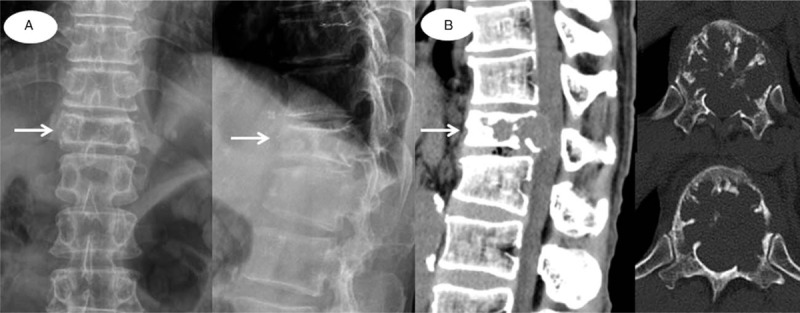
(A) Simple radiographs (anteroposterior and lateral) showed the decreased height of the T12 vertebral body. (B) CT images showed a burst fracture of the T12 vertebral body with an osteolytic lesion involving the vertebral body and pedicle, which is termed a “mini-brain appearance”. CT = computed tomography.
